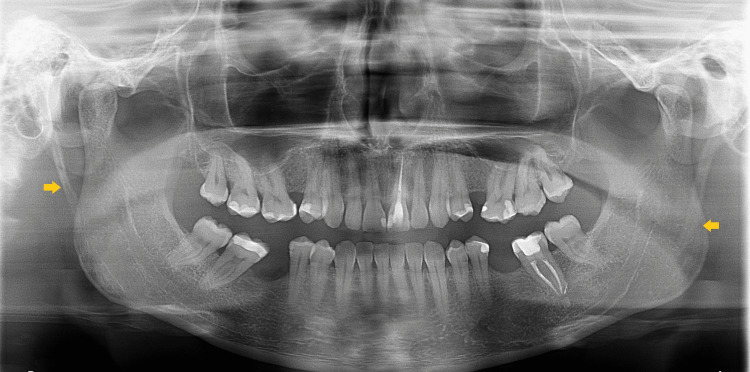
Panoramic radiograph showing a bilateral elongated styloid processes (yellow arrows).
